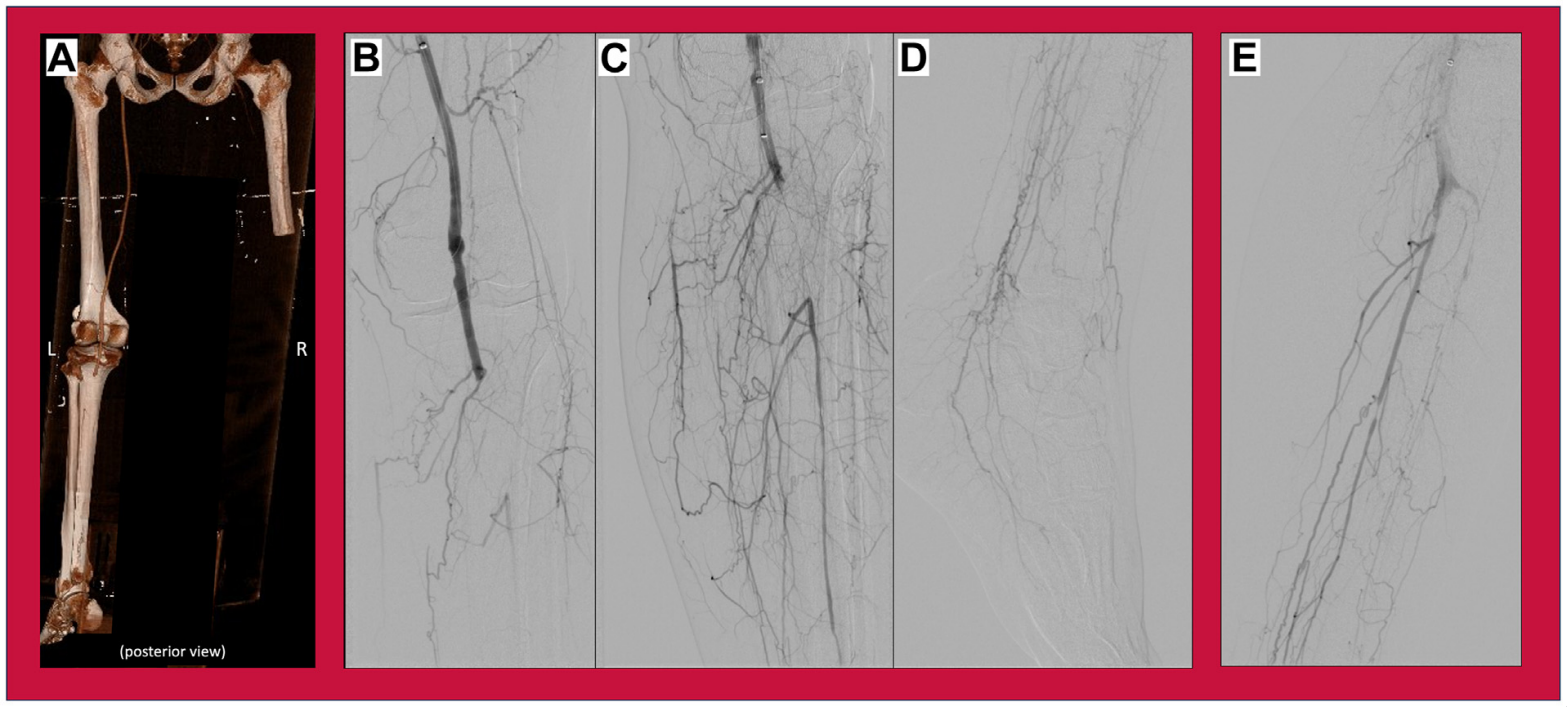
Computed tomography angiography (CTA) and angiogram, pre- and post-thrombectomy, during the third encounter, for left (contralateral to prior) acute limb ischemia. (A) Reconstructed three-dimensional CTA runoff posterior view showing occluded left popliteal artery proximal to stent; (B-D) Pre-intervention angiogram of the left leg; (E) Post-thrombectomy angiogram of the left leg.
